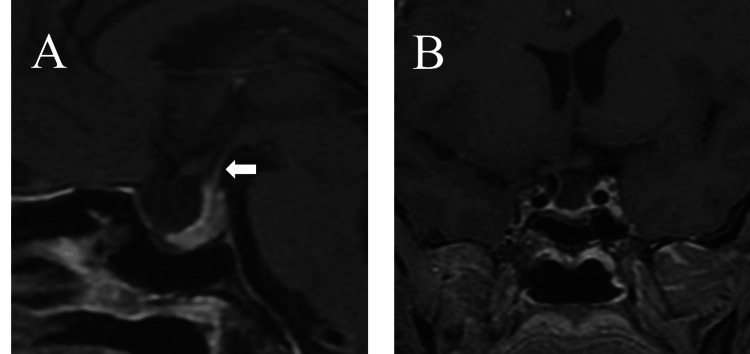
MRI of the pituitary gland reexamined seven days after surgery (A) Sagittal position on T1WI, complete pituitary stalk (white arrow); (B) coronal position on T1WI, complete resection of the tumor was observed
